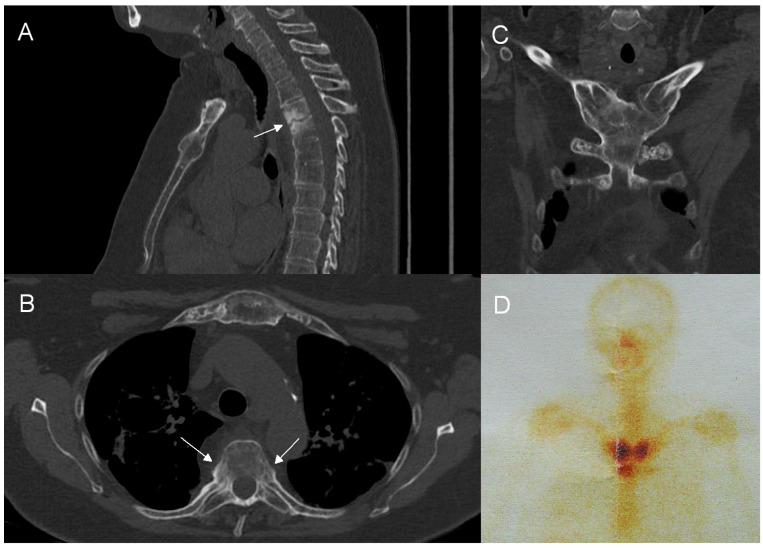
Concomitant involvement of the anterior chest wall and spine in a 54-year-old patient with SAPHO syndrome. CT shows the involvement of the thoracic spine (A) with an unstable Andersson lesion (arrow) and costovertebral joints ankylosis (arrows, (B)) and the hyperostosis and ankylosis of sternoclavicular joints, I costovertebral joints, and manubriosternal junction (C). An enlarged image from whole-body bone scintigraphy (D) shows an increased radiotracer uptake around sternoclavicular joints (‘bull’s head’ sign).
